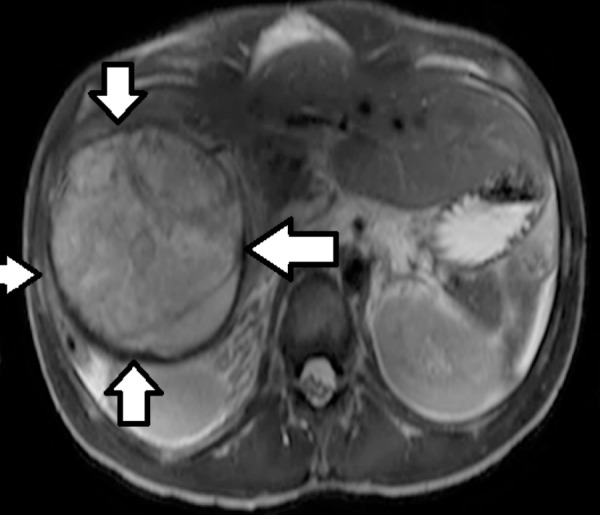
MRI showing cranial lesion of undifferentiated embryonal sarcoma of the liver MRI: Magnetic resonance imaging The cranial lesion of undifferentiated embryonal sarcoma of the liver has been highlighted by the arrows.
